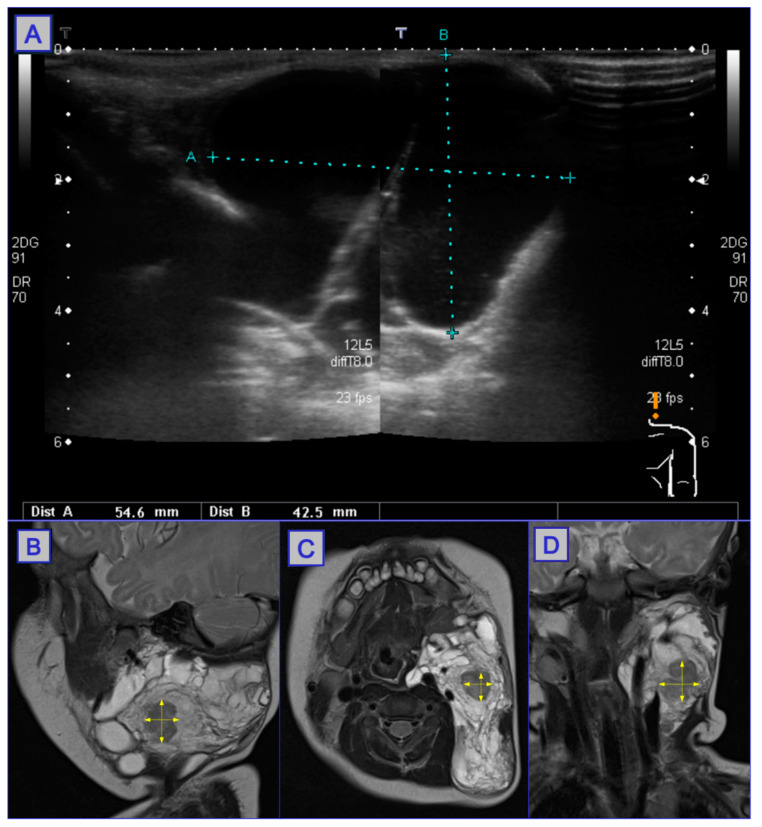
US imaging used to inspect the cystic hygroma (A), in which a large cystic lesion can be seen. MRI scanning (B–D) shows a considerable reduction in lesion size post-PDT. Reprinted with permission from ref. [88]. Copyright 2010 Photodiagnosis Photodyn. Ther.
